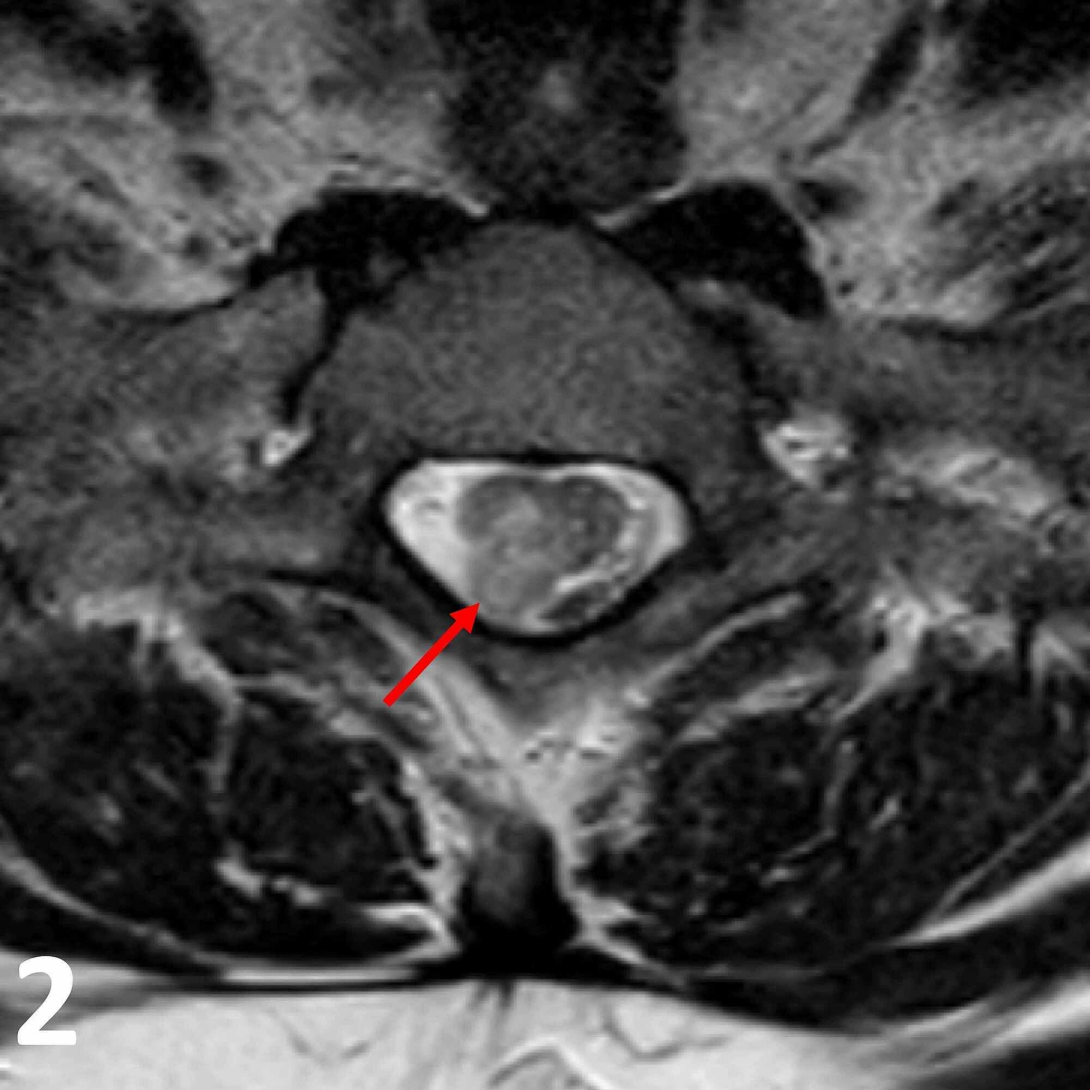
Axial T2 MRI image at the T1 vertebral level. Note the T2 heterogeneously hyperintense intradural lesion (red arrow) in the right posterolateral aspect of the spinal cord. CSF (T2 hyperintense signal) can be seen surrounding the spinal cord, signifying no spinal cord compression. CSF, cerebrospinal fluid
